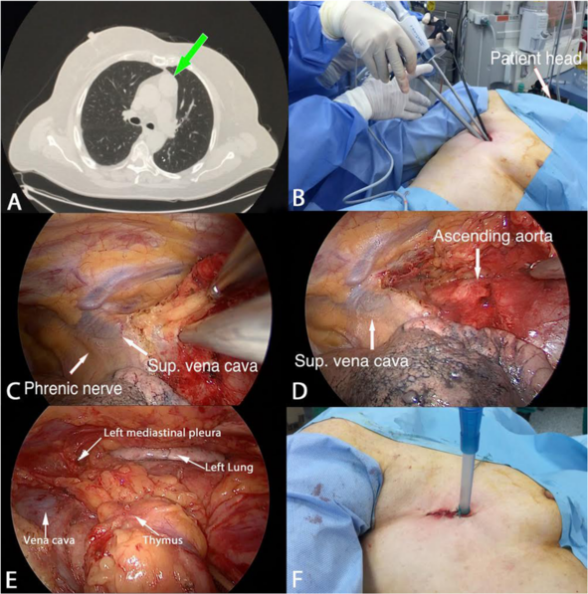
The chest computed tomography scan showed a 3,5 cm thymoma (Part a). Patient was placed in left lateral decubitus position, with the surgeon and the assistant standing on the posterior side (Part b). Dissection was landmarked by the superior border of phrenic nerve (Part c). The thymus was retracted superiorly and medially; the superior vena cava and the ascending aorta were skeletonised (Part d). Right side thymic and perithymic fatty tissue dissection was completed and left lung visible (Part e). Closure of right incision with a chest drainage (Part f)
